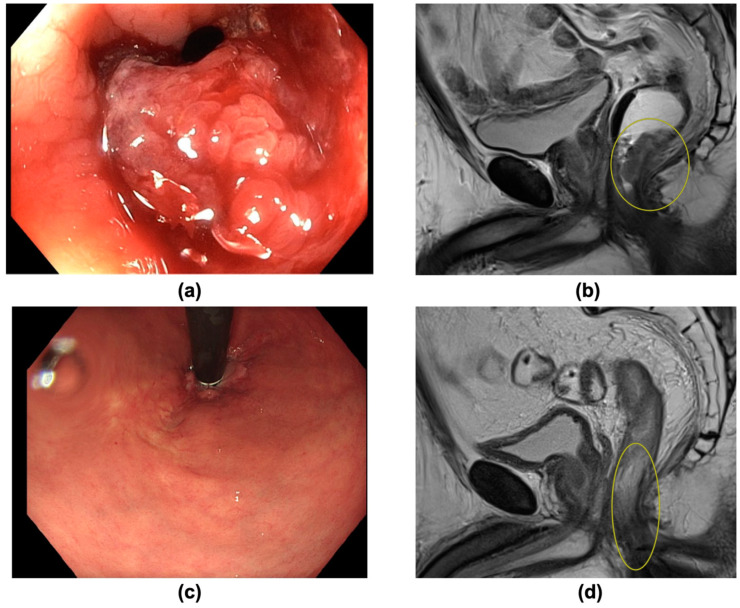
(a–d): A 63-year-old patient with distal rectal carcinoma (cT4 cN0 cM0) was undergoing Total Neoadjuvant Therapy (TNT), obtaining clinical complete response (cCR). A non-operative management was chosen. There was no recurrence within an 18-month follow-up. (a) Image of endoscopy prior to treatment, showing a vulnerable ulcerated tumor. (b) Pre-treatment sagittal T2-weighted MRI scan, picturing a distal T4 tumor (yellow circle) in minimal distance from the anal sphincter. (c) Endoscopy 8 weeks after TNT, showing a macroscopic good response with a residual scar. (d) Post-treatment sagittal T2-weighted MRI scan confirming clinical complete response 8 weeks after TNT (yellow circle: former tumor bed).
